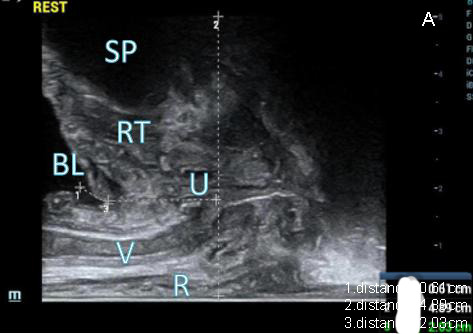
Midsagittal plane obtained by biplanar transrectal ultrasound at rest: normal pelvic floor image.
